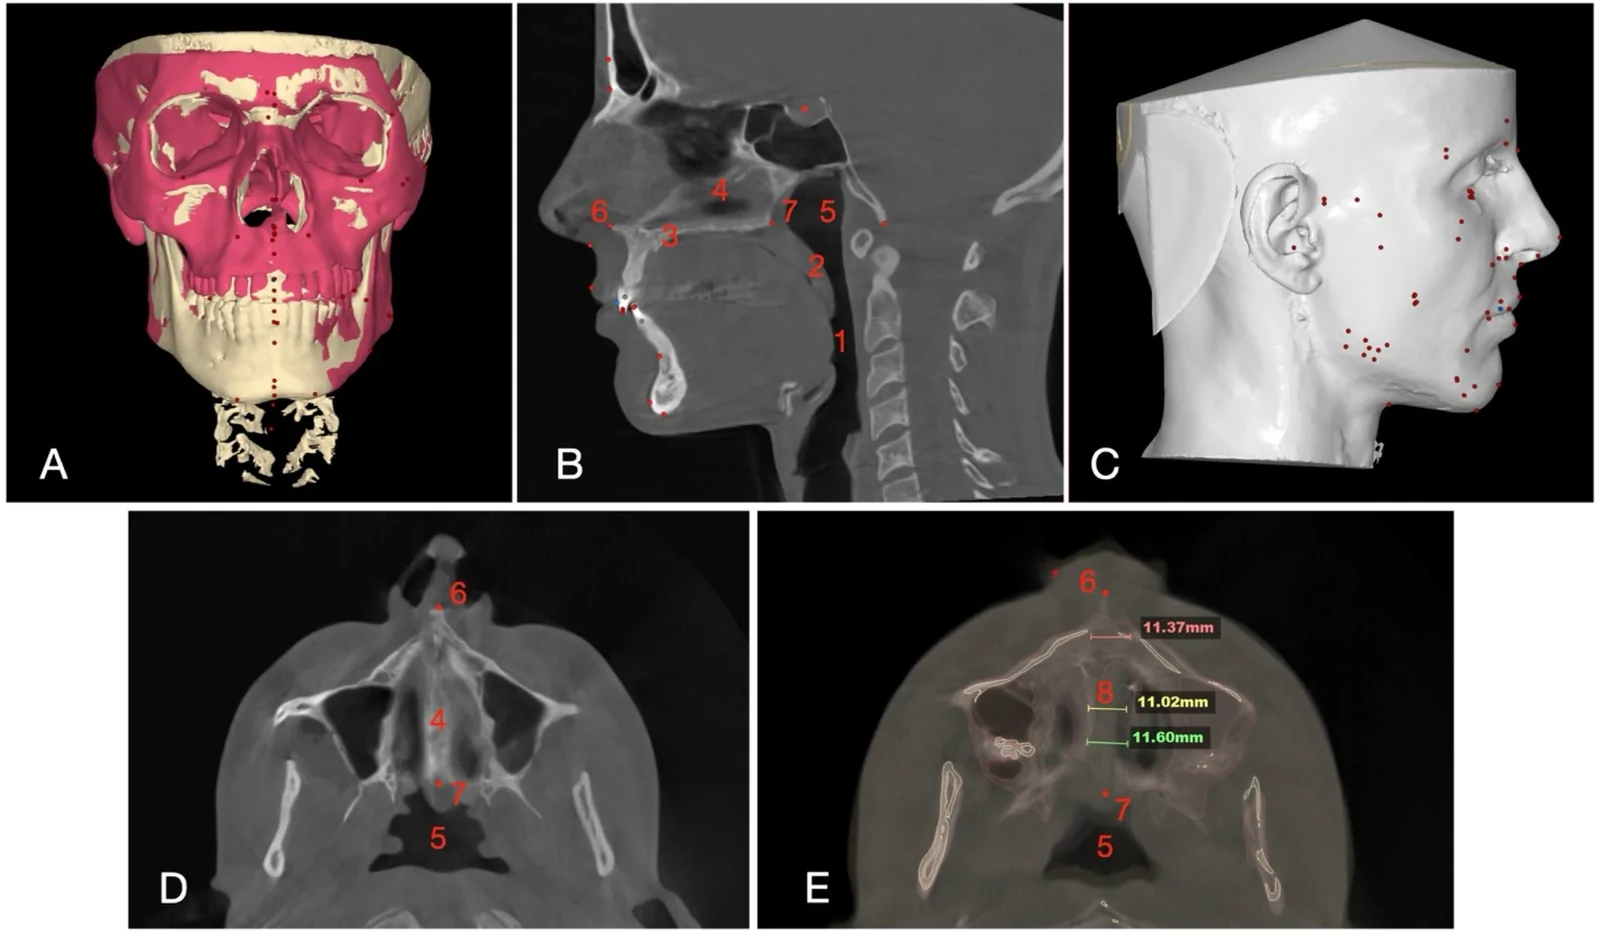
3-D cBCT measurements: (A) superimposition of the 3D cBCT volumes from before (beige) expansion and after the completion of expansion (magenta), (B) landmark allocation [1 – oropharyngeal airway space, 2 – soft palate and uvula, 3 – palatal plane, 4 – nasal septum sagittal view, 5 – nasopharyngeal airway space, 6 – anterior nasla spine (ANS), 7 – posterior nasal spine (PNS)], (C) soft tissue mesh landmarks, (D) axial view of the palatal plane with the nasal septum base before expansion, (E) axial view of the palatal view after completion of expansion with the vomer (8) as a reference of the nasal septum and three measured intermaxillary width measurements (anterior, medial, and posterior).
